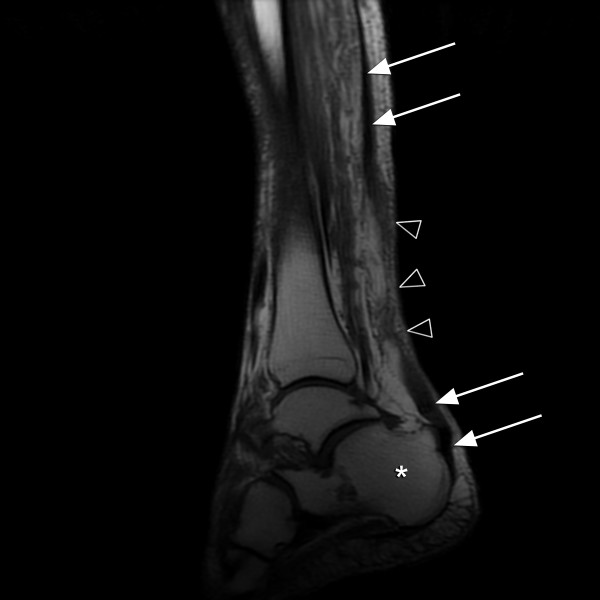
T1-weighted image of the patient's right heel. T1 images do not amplify the brightness of low-density tissue, and therefore do not risk obscuring pathologic findings in more dense tissues, such as tendon and bone. On the image above, arrows follow the complete rupture of the Achilles tendon. Note how the distal end lays 'floppy' over the superior calcaneus (asterisk). The open arrow-heads note intervening inflammation and soft-tissue edema.
